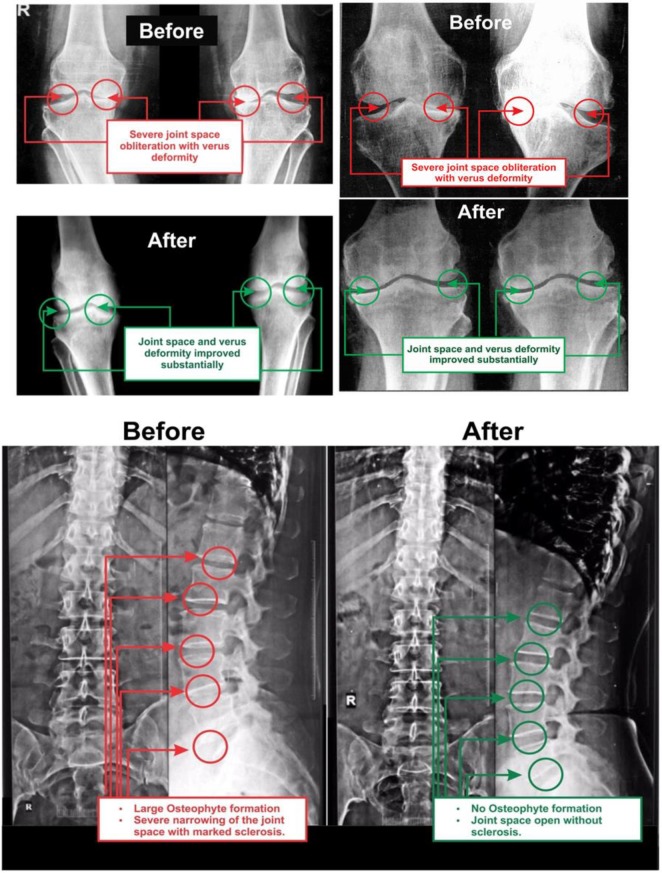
AP views of x-ray for knee-joint and lumbar vertebrae before and after the treatment
